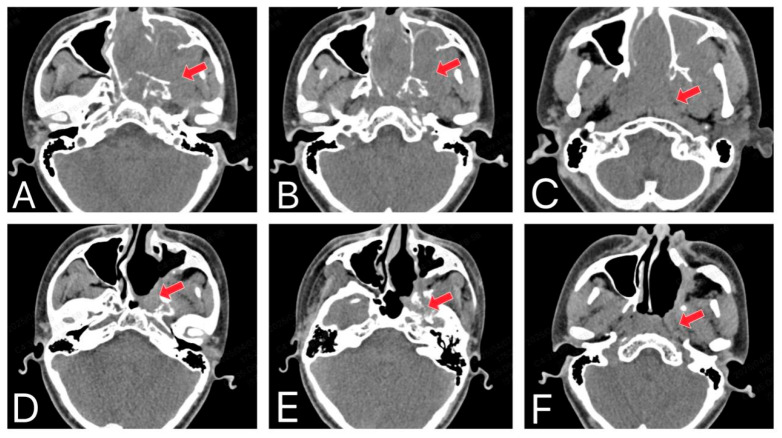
The invasive nature and recurrence of JNA. (A–C) CT shows that the original JNA originates from the posterolateral wall of the nasal cavity, near the pterygopalatine fossa region, and invades the surrounding bone. (D–F) The CT shows a recurrent JNA after surgery (The red arrow in the image indicates the lesion site).
